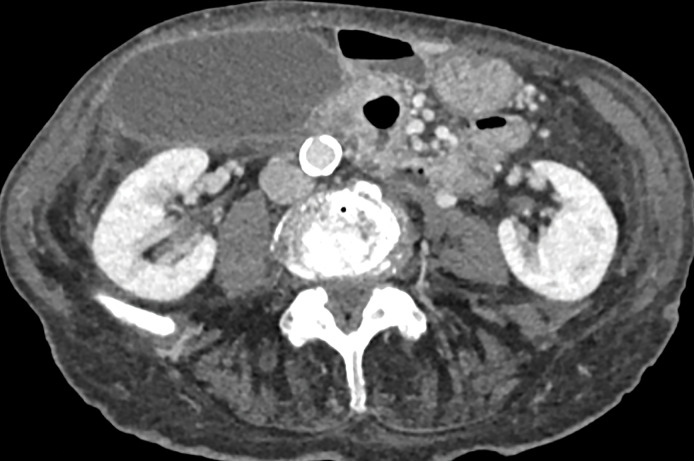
Axial image of a CT scan during the portal phase showing distended gallbladder in horizontal position, outside the liver bed, with no dense gallstones and mild pericholecystic fluid.
